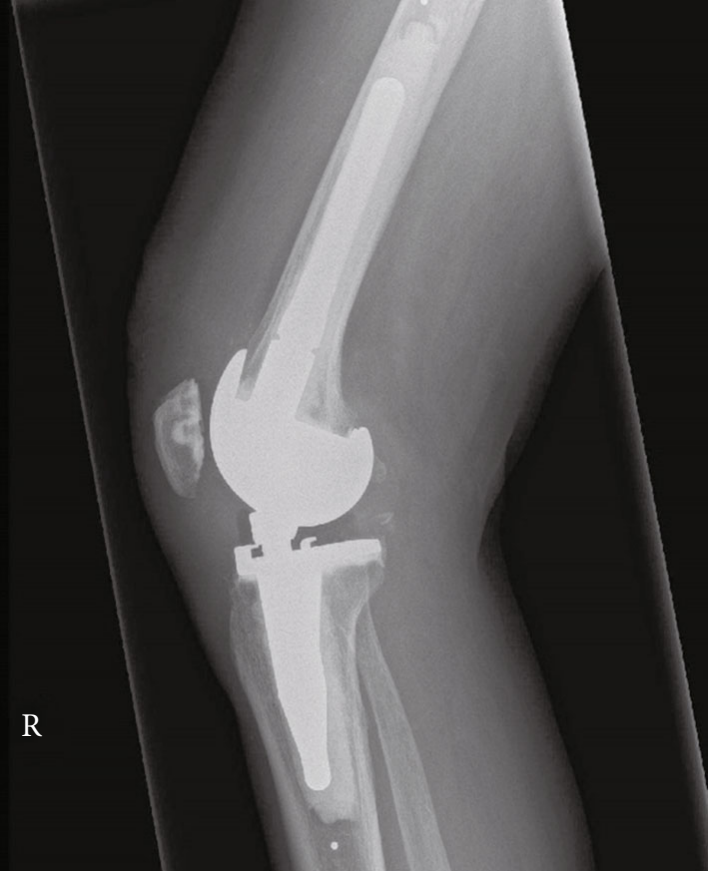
Postoperative radiographs showing the lateral view of the right knee.
